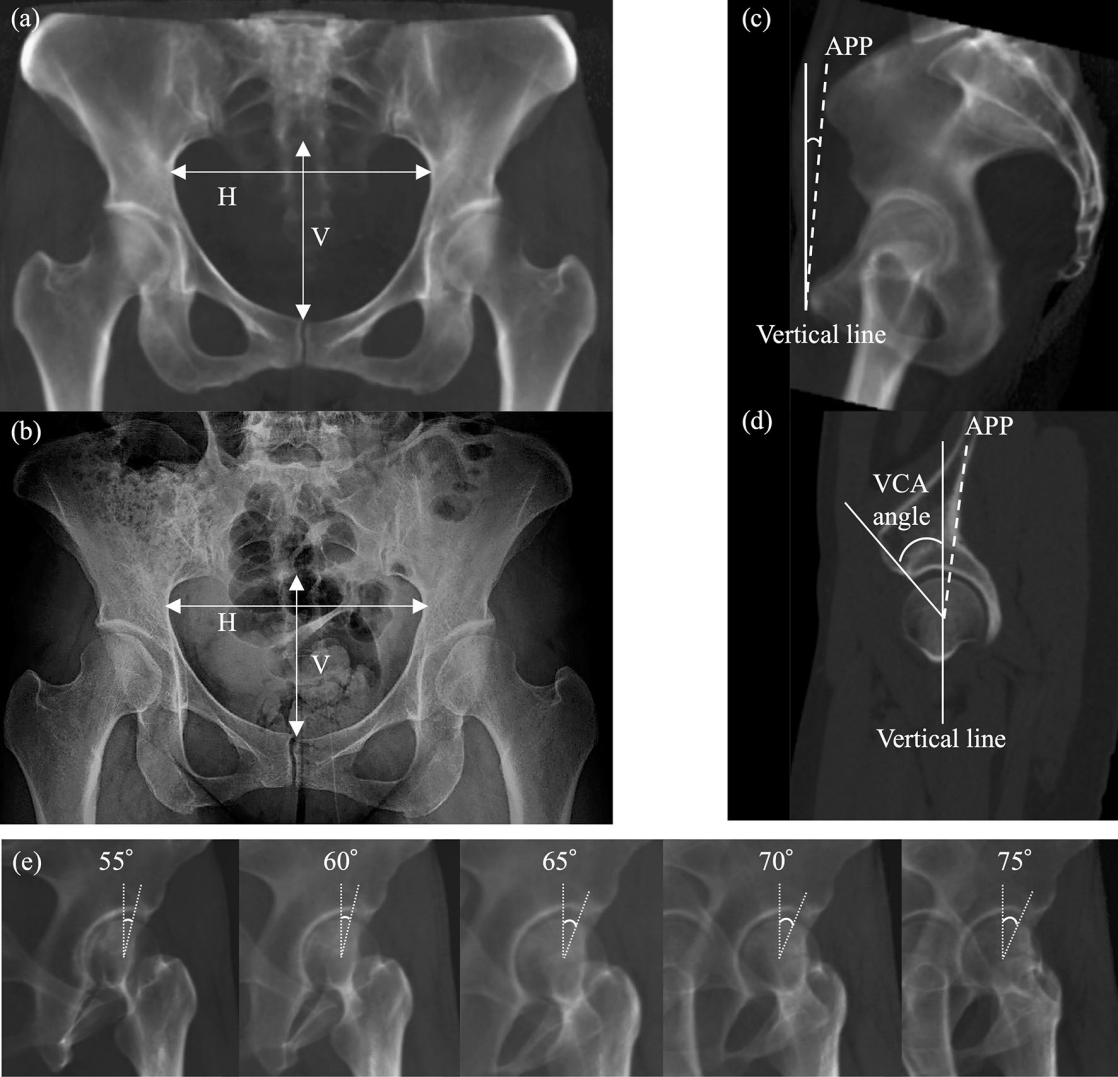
DRR image (a) and standing radiograph (b). Adjust the pelvic inclination (c) so that the vertical-to-horizontal ratio of the pelvic foramen is the same between (a) and (b). The arrows indicate the vertical distance between the bilateral sacroiliac joints and pubic symphysis and the maximum horizontal diameter of the pelvic foramen. The pelvic inclination was defined as the angle between the solid line (z-axis) and the dotted line (APP). (d) VCA angle was measured as the angle between the vertical line and a line connecting the femoral head center and the anterior end of the acetabulum after adjustment to the standing position. The dotted line indicates APP. (e) DRR image of the FP radiograph (rotating AP image 65° in the axial plane), ± 5° and ± 10°relative to the FP radiograph (rotating AP image 55°, 60°, 70°, 75°in the axial plane). Indicates a change of sourcil edge with pelvic rotation. The ACEA was measured for each DRR image. *DRR* Digitally reconstructed radiography, *APP* Anterior pelvic plane, *VCA* Vertical-center-anterior, *FP* False profile, *AP* Anterior–posterior, *ACEA* Anterior center edge angle.
